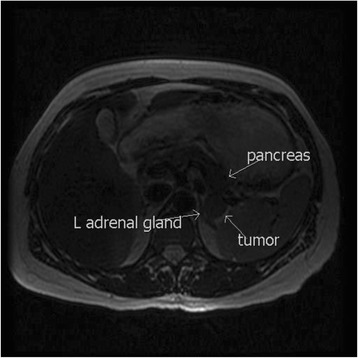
Magnetic resonance imaging demonstrated hyperintense solid lesion 2 cm in diameter localized in the area of the pancreatic tail
